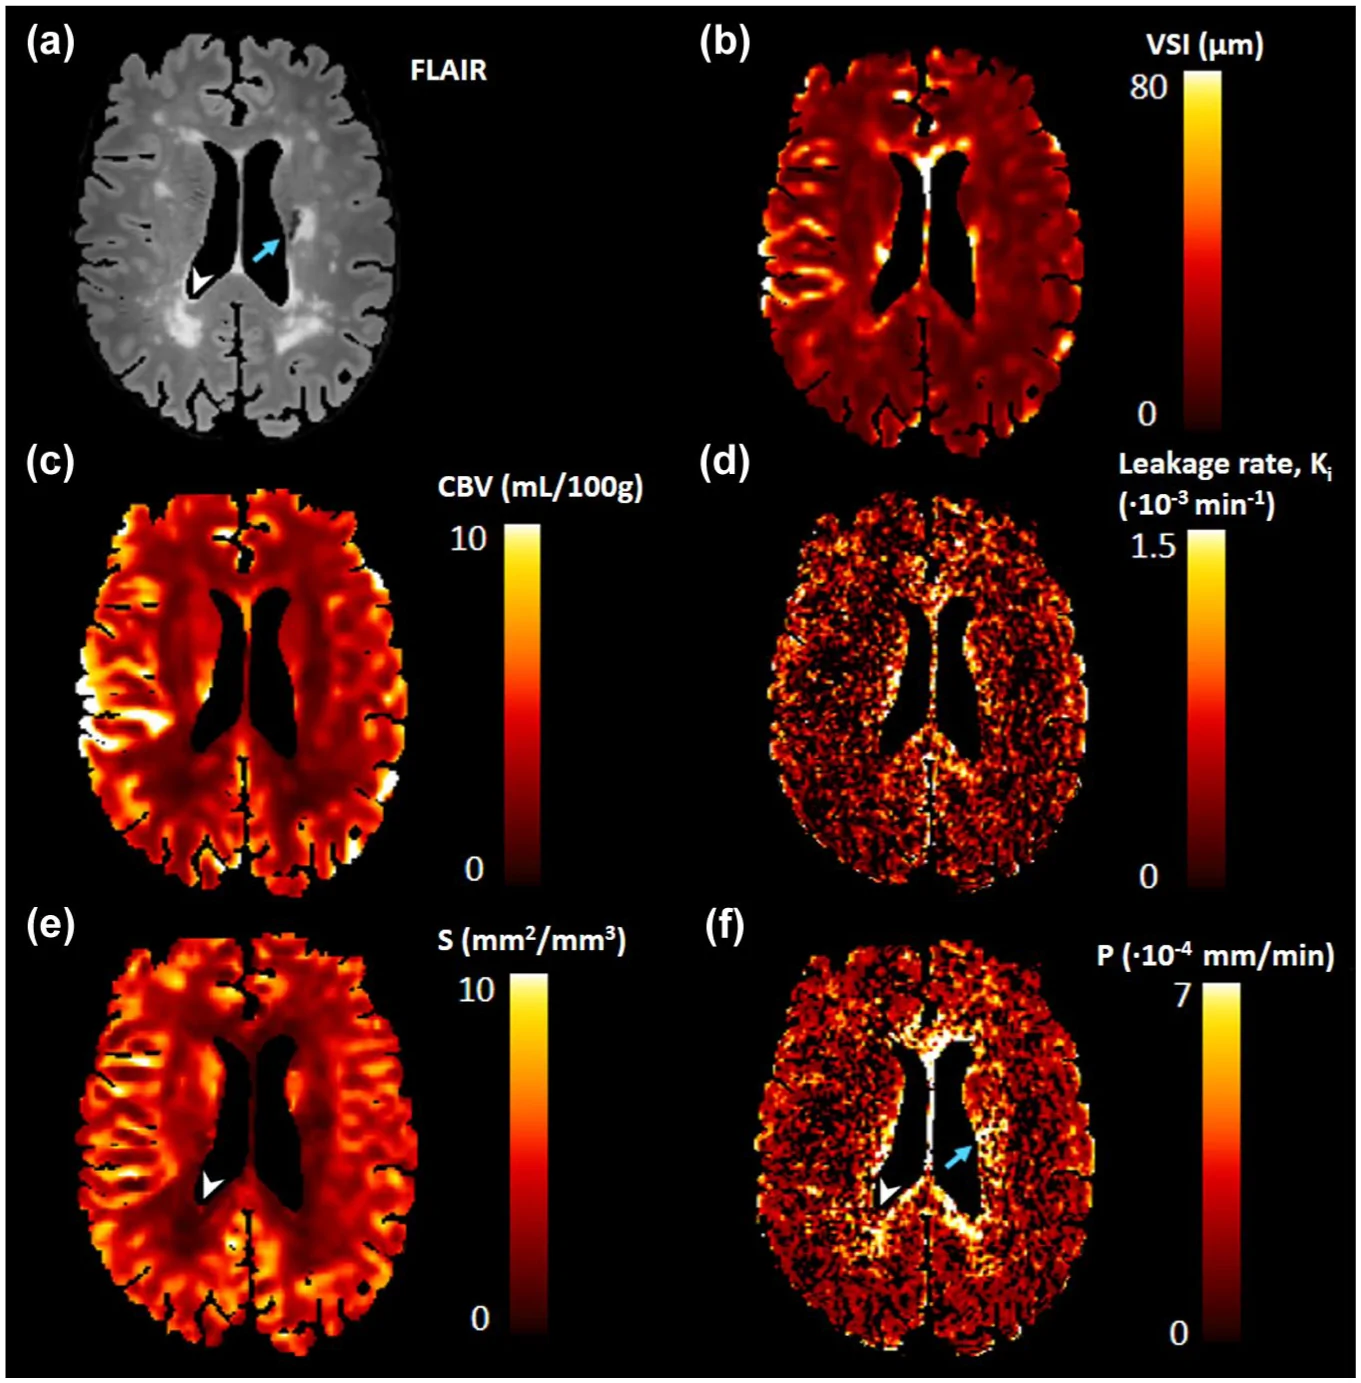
Brain maps for a cSVD patient (60 years old, male) showing: (a) white matter hyperintensities and a lacunar infarct on a T2 FLAIR image, (b) VSI, (c) CBV, and (d) blood–brain barrier leakage rate (*K_i_*), which can be split up in (e) vascular surface area (*S*) and (f) intrinsic vascular permeability (*P*). The white arrowhead shows decreased *S* in white matter hyperintensities, increasing conspicuousness on *P*, but not *K_i_* maps. The blue arrow points to an approximately 7-year-old infarct, whose surroundings show increased *P*, but not *K_i_*. CBV: cerebral blood volume; FLAIR: fluid-attenuated inversion recovery; VSI: vessel size index.
